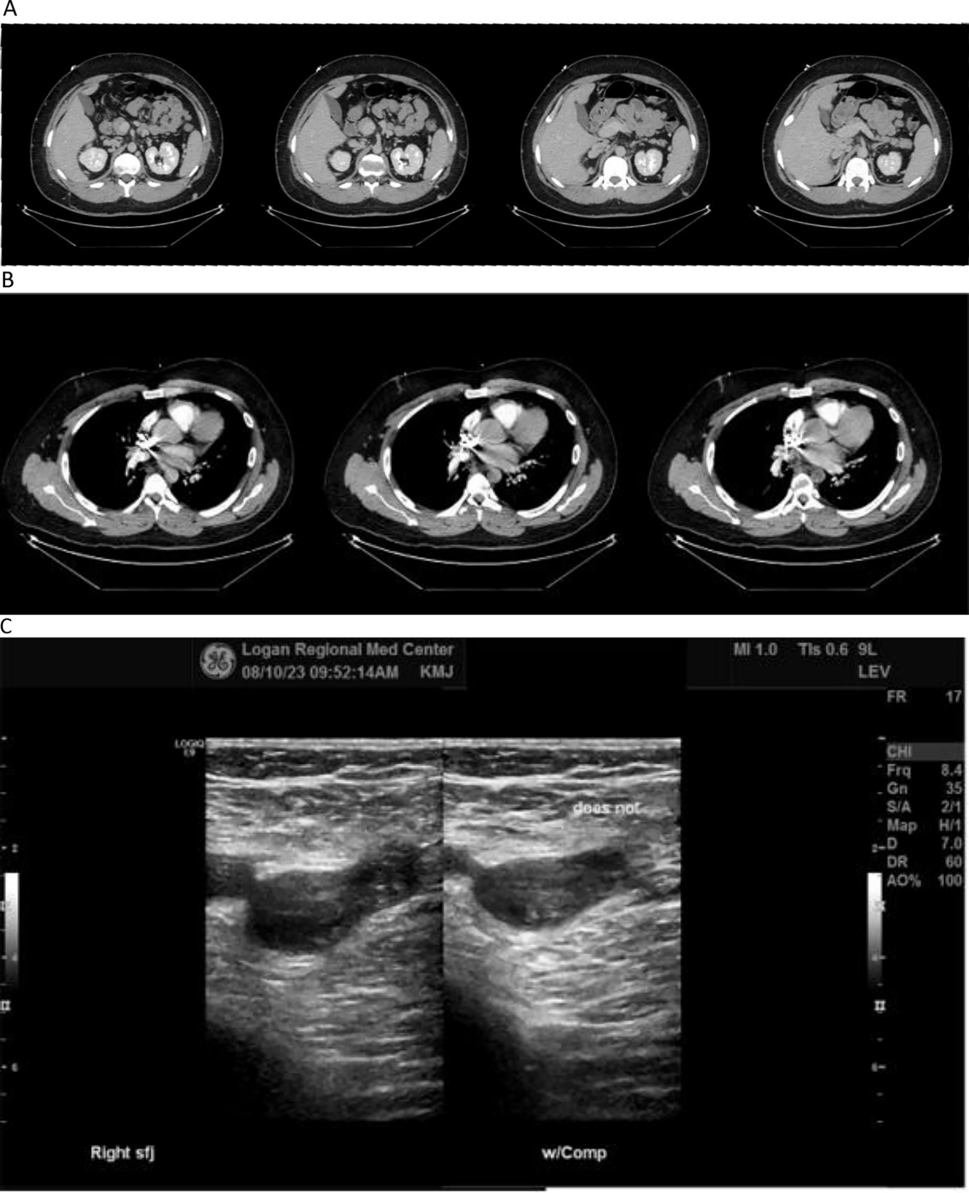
(A–C) diagnostic imaging. (A) CT of abdomen with contrast. (B) CT of chest with contrast. (C) Venous doppler ultrasound of right leg. Contrast-enhanced CT scans of patient's abdomen (A) and chest (B) showing evidence of pulmonary emboli in left central pulmonary arteries, and agenesis of the inferior vena cava with extensive collateralization. Venous Doppler ultrasound of the right lower extremity (C) showing thrombus present from the common femoral vein distally into the popliteal vein extending into the greater saphenous vein.
